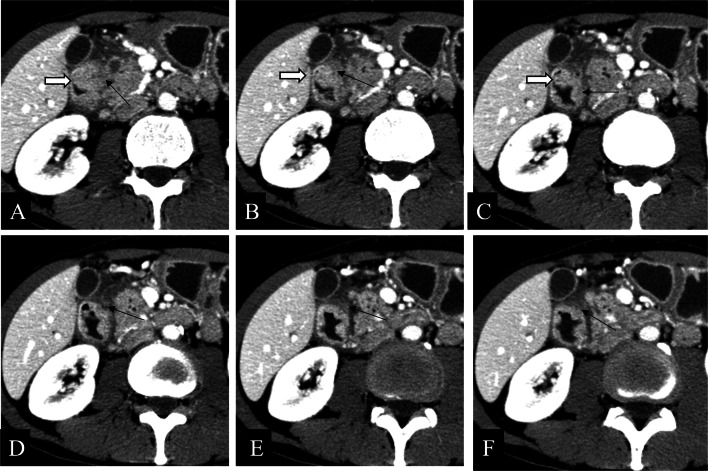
Groove pancreatitis in a 44-year-old male. a–f Axial contrast-enhanced CT images showed a sheetlike hypoattenuating mass (black arrows) in the pancreaticoduodenal groove and separated from the pancreatic head and the duodenum, with thickening of adjacent medial wall of the duodenum. a–c The final pathology demonstrated a 2.7 cm sized heterotopic pancreas in the duodenum (white arrows)
